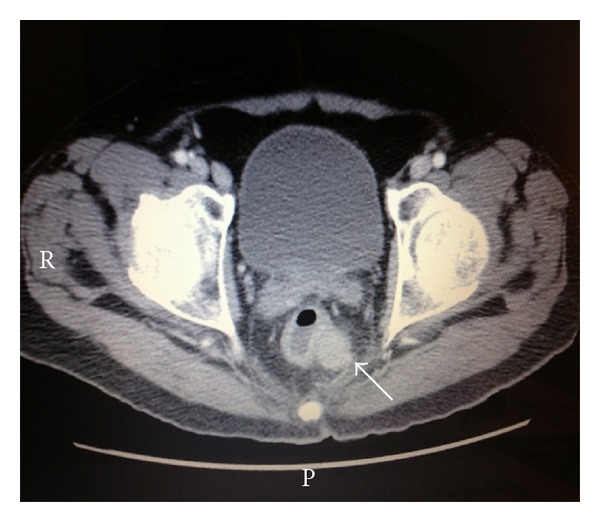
Staging CT scan showed a left perirectal soft tissue mass which is 2.0 × 2.2 cm in diameter.
